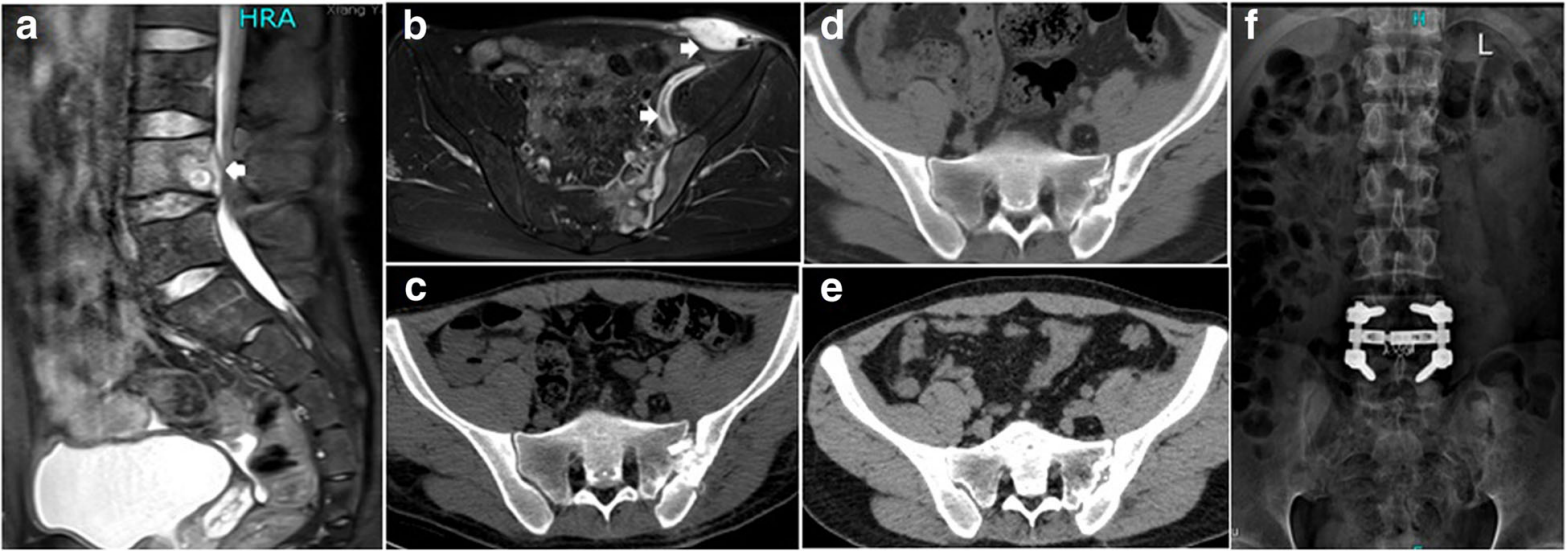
MRI and CT of patient NO.11 pre- and post-operation of 3,6 and 12 months and X-ray of 12 months after surgery. a L4 vertebra was eroded and epidural sac was compressed (b)Arrow indicates a inguinal abscess with a sinus track attaching to iliopsoas abscess. c-e)Procession of sacroiliac joint fusion at 3,6 and 12 months postoperation. Solid joint fusion was found within 12 months postoperation. (f)X-ray indicated solid joint fusion and good instrumentation
